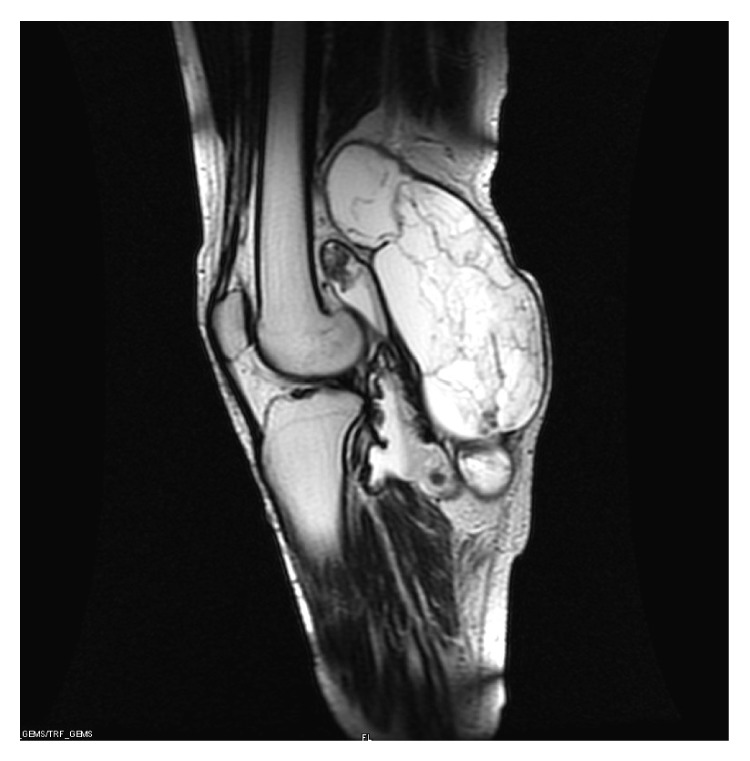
The sagittal section of the popliteal mass on MRI showed the enormous mass.
